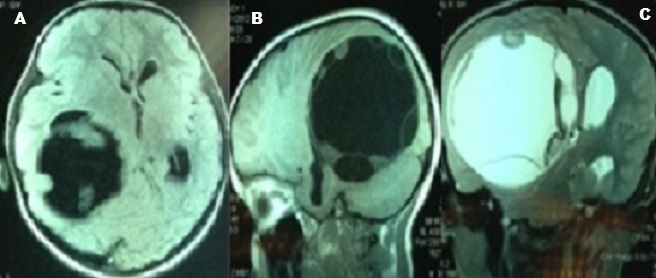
A) axial T1 weighted MR image shows a large hypointense parietal cystic lesion; B) sagittal T1 weighted MR image shows daughter cysts; C) coronal T2 weighted MR image shows mass effect and shift of the midline structures
